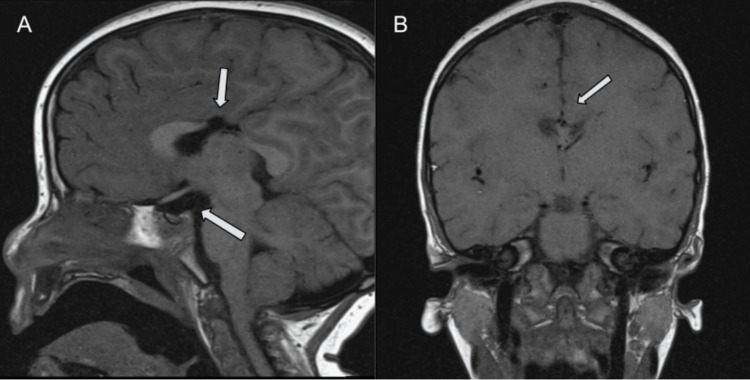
Segmental agenesis of the corpus callosum and pituitary hypoplasia A) Sagittal T1-weighted image (T1WI) shows an intact rostrum, intact genu, absent callosal body, intact isthmus, and intact splenium. Note the small pituitary volume (6.2 x 1.5 x 6.7mm), with a lack of normal T1WI hyperintensity of the neurohypophysis.  B) Coronal T1WI confirms absent commissural fibers bridging the midline.
